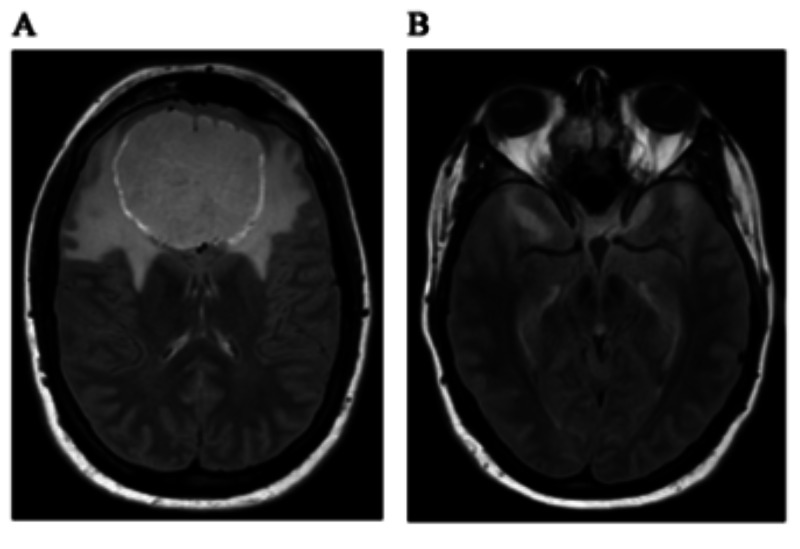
Pre-Op CT Contrast-enhanced T2 flair magnetic resonance imaging revealing significant cerebral edema involving the bifrontal lobes (A) and temporal horns (B).
